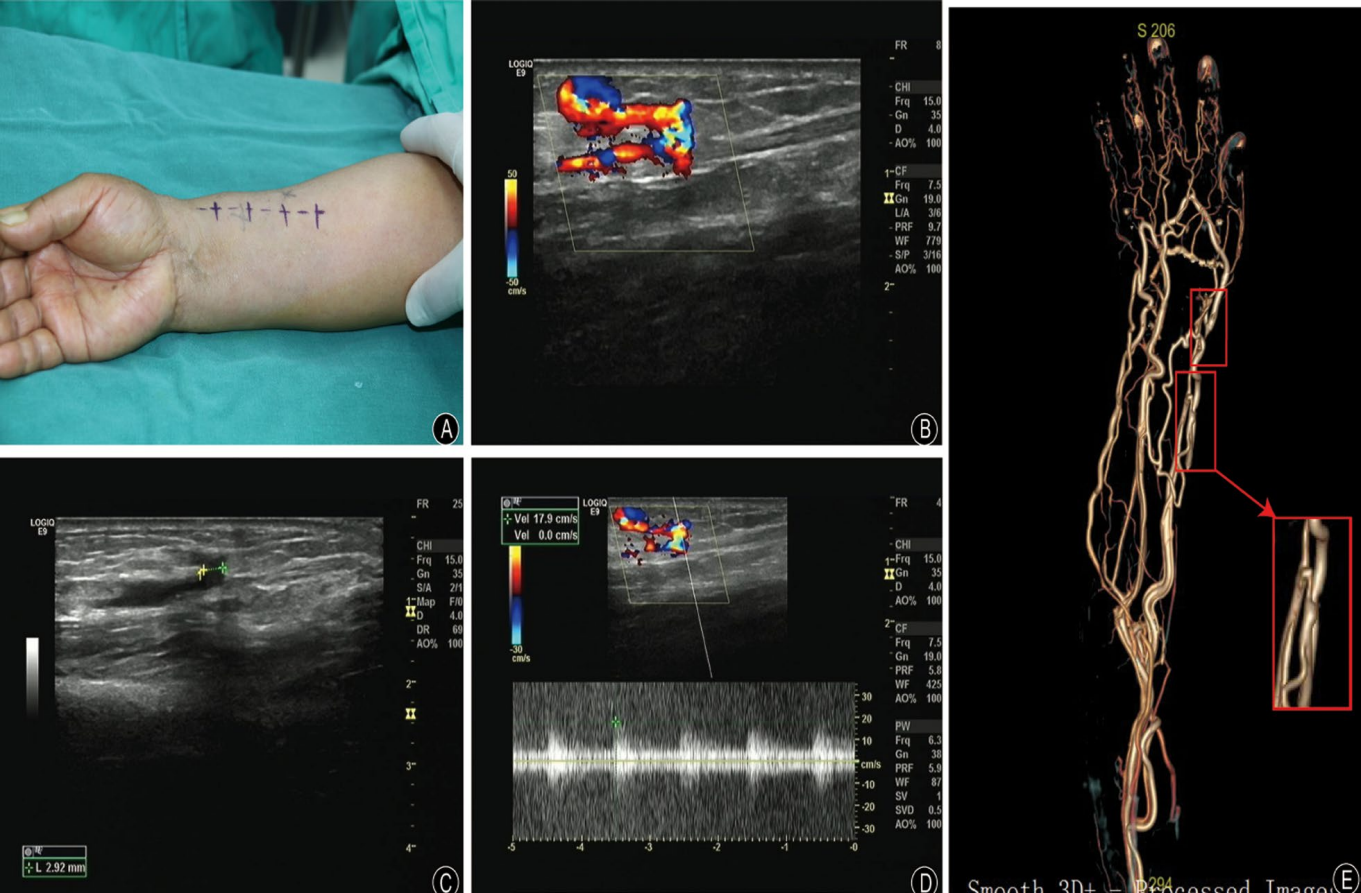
Preoperative appearance photos and images. A The appearance photo showed a slight bump and palpable thrill of the forearm. Doppler ultrasound showed: B continuous turbulent flow between radial artery and vein; C the diameter of the fistula neck; D arterial spectrum of the fistula. E Upper extremity CT angiography revealed AVF formation between the right radial artery and the adjacent vein around the right wrist. In addition, the continuity of the radial artery was lost just distal to the AVF on the image
